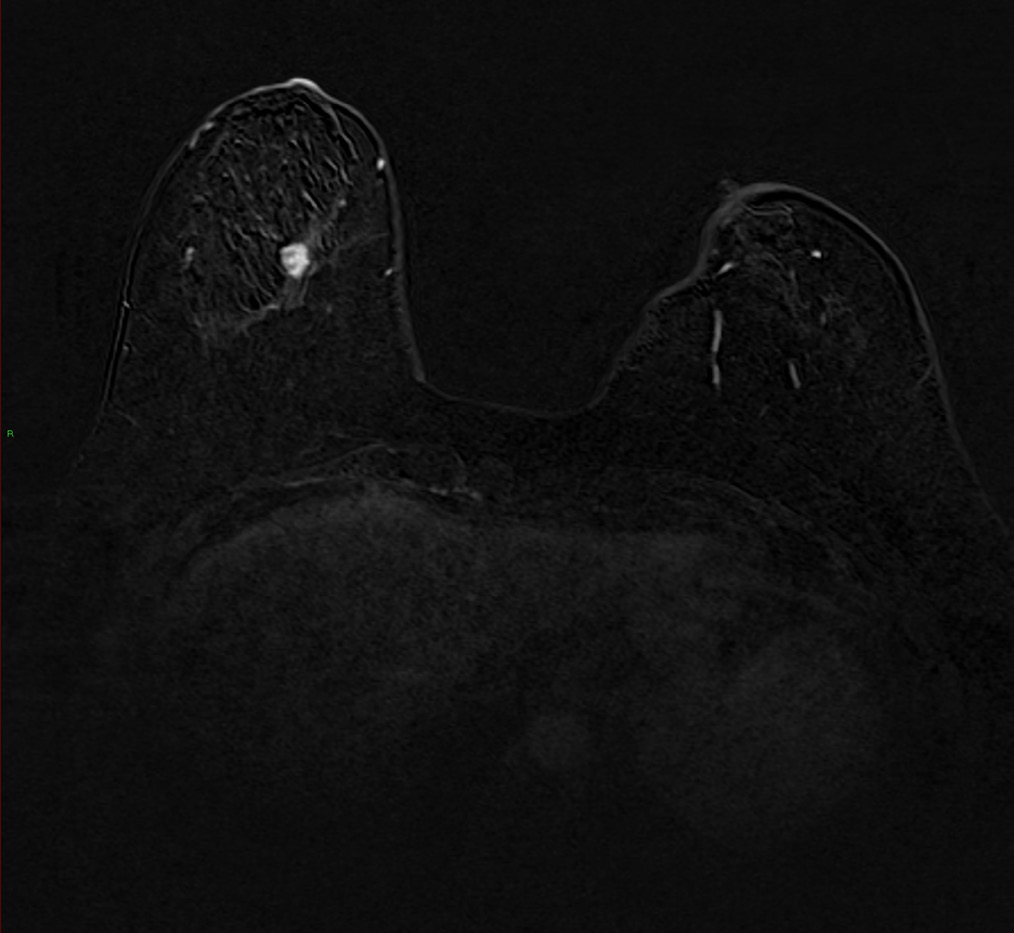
MRI post-contrast subtracted axial image of the breast. There is a 12
